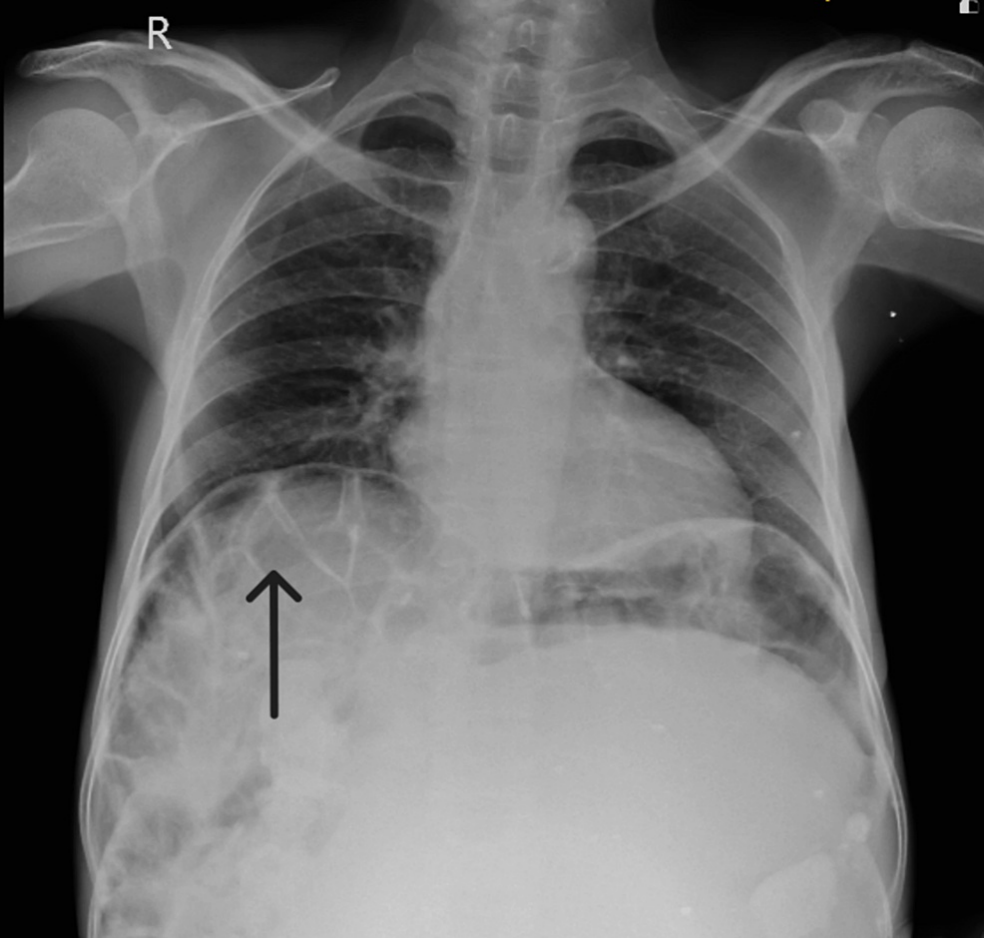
Chest X-ray (posteroanterior view) showing the elevated right hemidiaphragm with interposition of the transverse colon between the diaphragm above and liver below. Chilaiditi's sign (black arrow).
